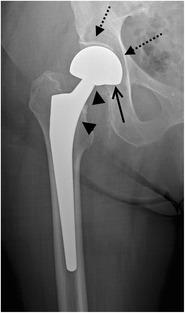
AP radiograph. Cementless bipolar hemiarthroplasty. The femoral stem with a fixed head (*arrowheads*) articulates with a polyethylene lined metal cup (*arrow* indicates position of the radiolucent polyethylene), which articulates with the native acetabulum (*dotted arrow*)
